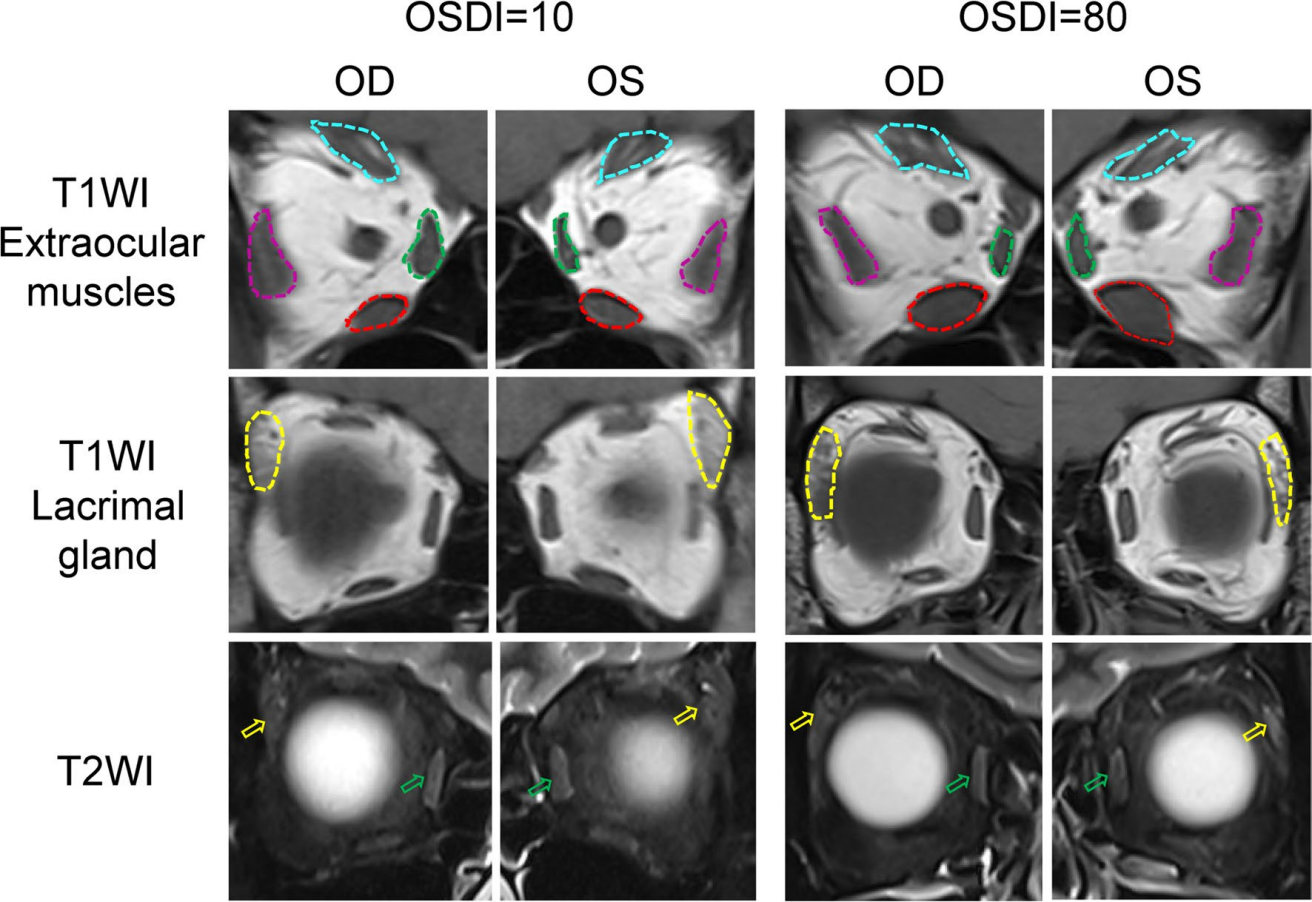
MRI scans of lacrimal gland and extraocular muscles. The blue dashed line represents the area of the superior rectus, the green dashed line represents the area of the medial rectus, the red dashed line represents the area of the inferior rectus, the purple line represents the lateral rectus, and the yellow dashed line represents the area of the lacrimal gland. The yellow arrow represents the lacrimal gland and the green one represents the medial rectus. Abbreviation: OSDI, Ocular Surface Disease Index
